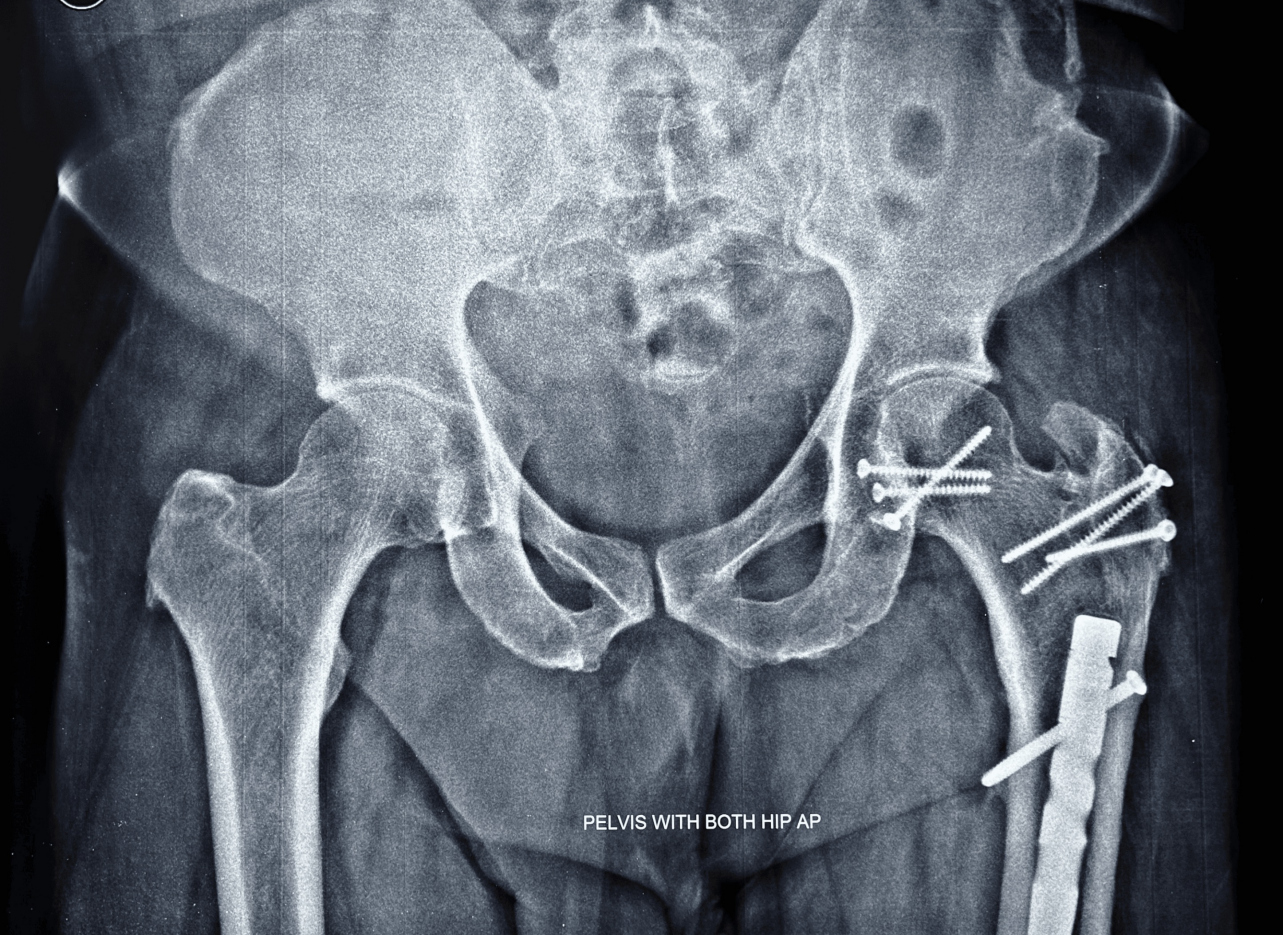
At 96 months follow-up, X-ray of both hips anteroposterior (AP) view – no signs of avascular necrosis (AVN) or post-traumatic arthritis (PTA)
